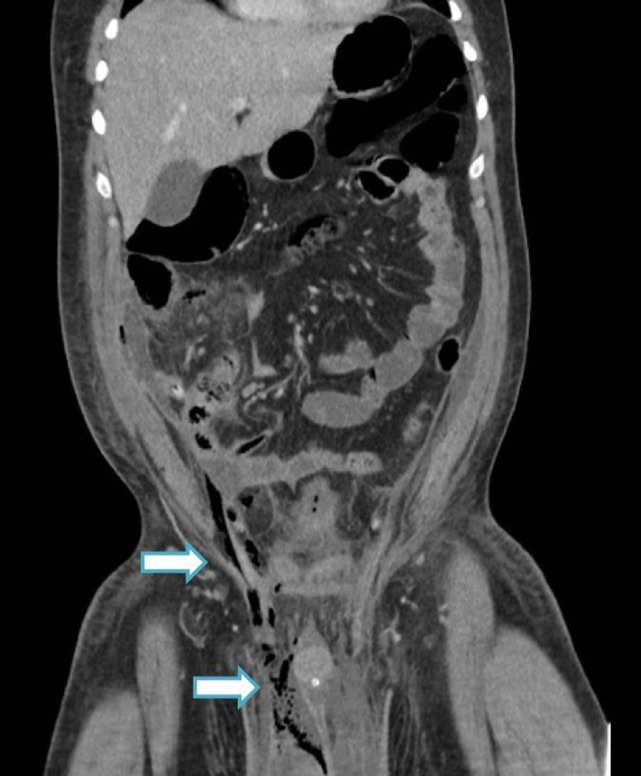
CT image (coronal slice) showing gas tracking from the right iliac fossa, down the spermatic cord into the scrotum.
